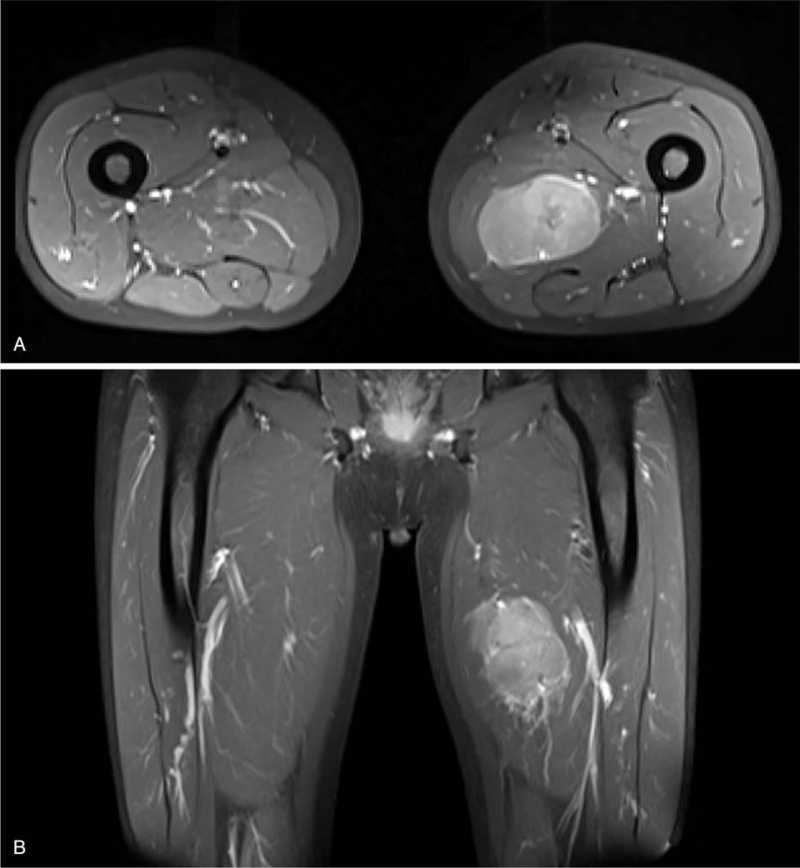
Axial (A) and coronal (B) T1 views of an MRI of the lesion in the context of the left medial thigh muscle. MRI = magnetic resonance imaging.
